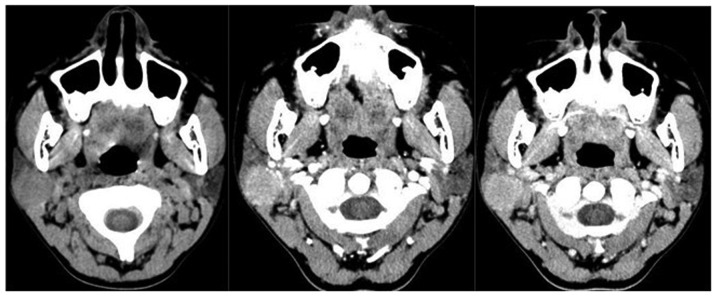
CT of a 14-year-old female patient showing a round soft tissue mass invading the deep lobe. The unclear margin between the sternocleidomastoid and the mass was more apparent following CT enhancement. CT, computed tomography.
